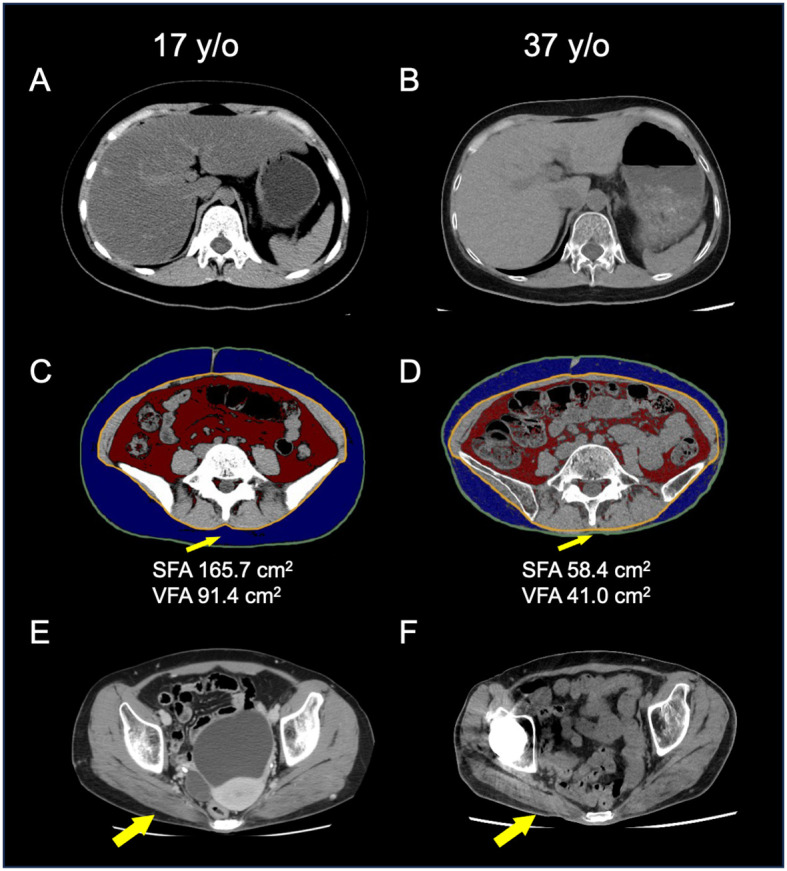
Computed tomography (CT) images demonstrating changes in fat distribution and hepatic steatosis. (A, B) Axial CT images at the upper abdominal level obtained at 17 (A) and 37 (B) years of age, showing improvement in hepatic steatosis. (C, D) Axial CT images at the umbilical level obtained at 17 (C) and 37 (D) years of age. Subcutaneous fat area (SFA) and visceral fat area (VFA) are shown. Both SFA and VFA are reduced at 37 years compared with 17 years. In panel D, an arrow indicates a reduction in subcutaneous fat in the lower back region. (E, F) Axial CT images at the pelvic level obtained at 17 (E) and 37 (F) years of age. Subcutaneous fat in the gluteal region is reduced at 37 years, as indicated by arrows.
